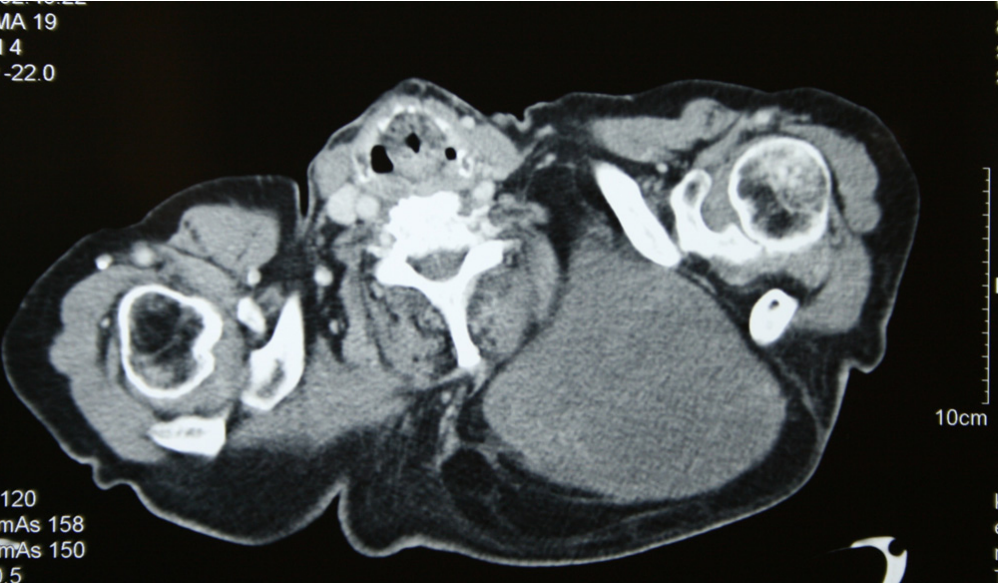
Chest CT scan of the patient
